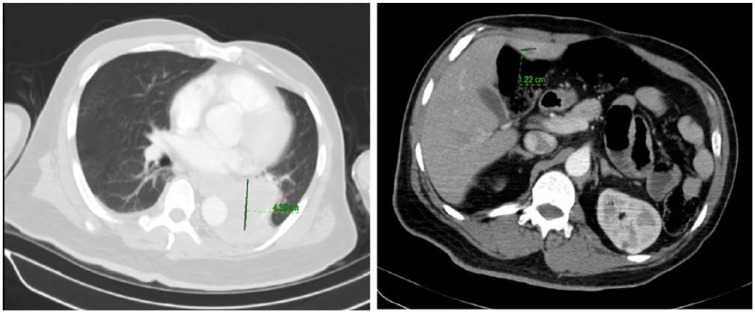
Computed tomography demonstrating large hilar lung mass and liver nodules.
